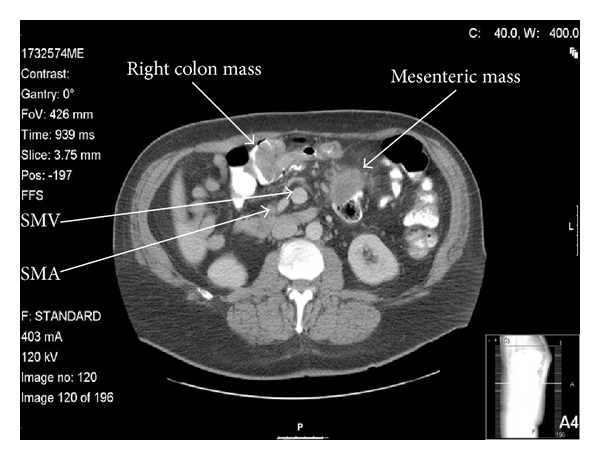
Right colon and mesenteric masses on CT.
